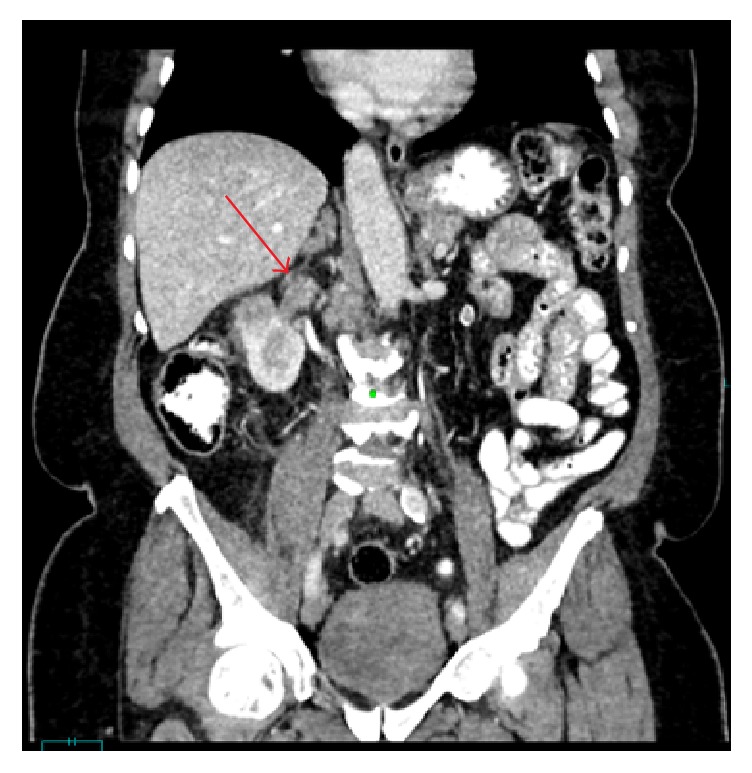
Contrast enhanced computed tomography coronal image demonstrates tumoural thrombus within the right renal vein (red arrow).
